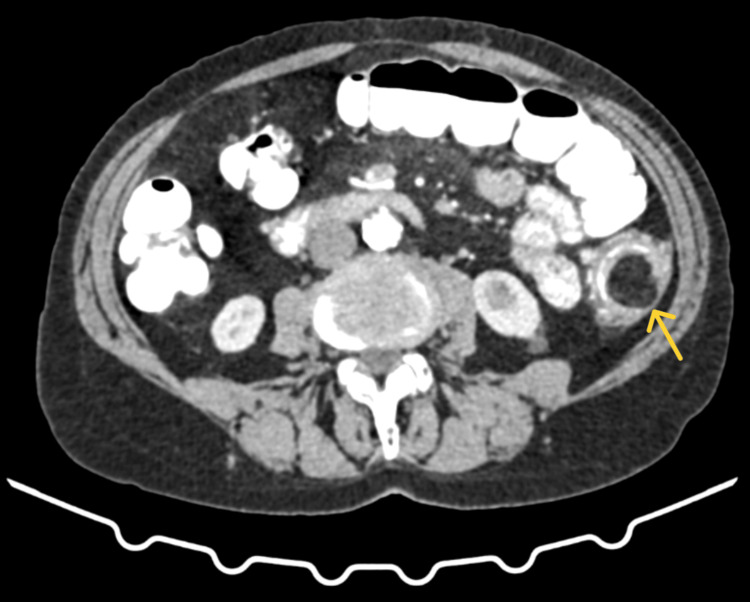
Contrast-enhanced abdominal computed tomography. Axial plane. A submucosal lipoma (yellow arrow) of 2.6 centimeters at the level of the descending colon.
